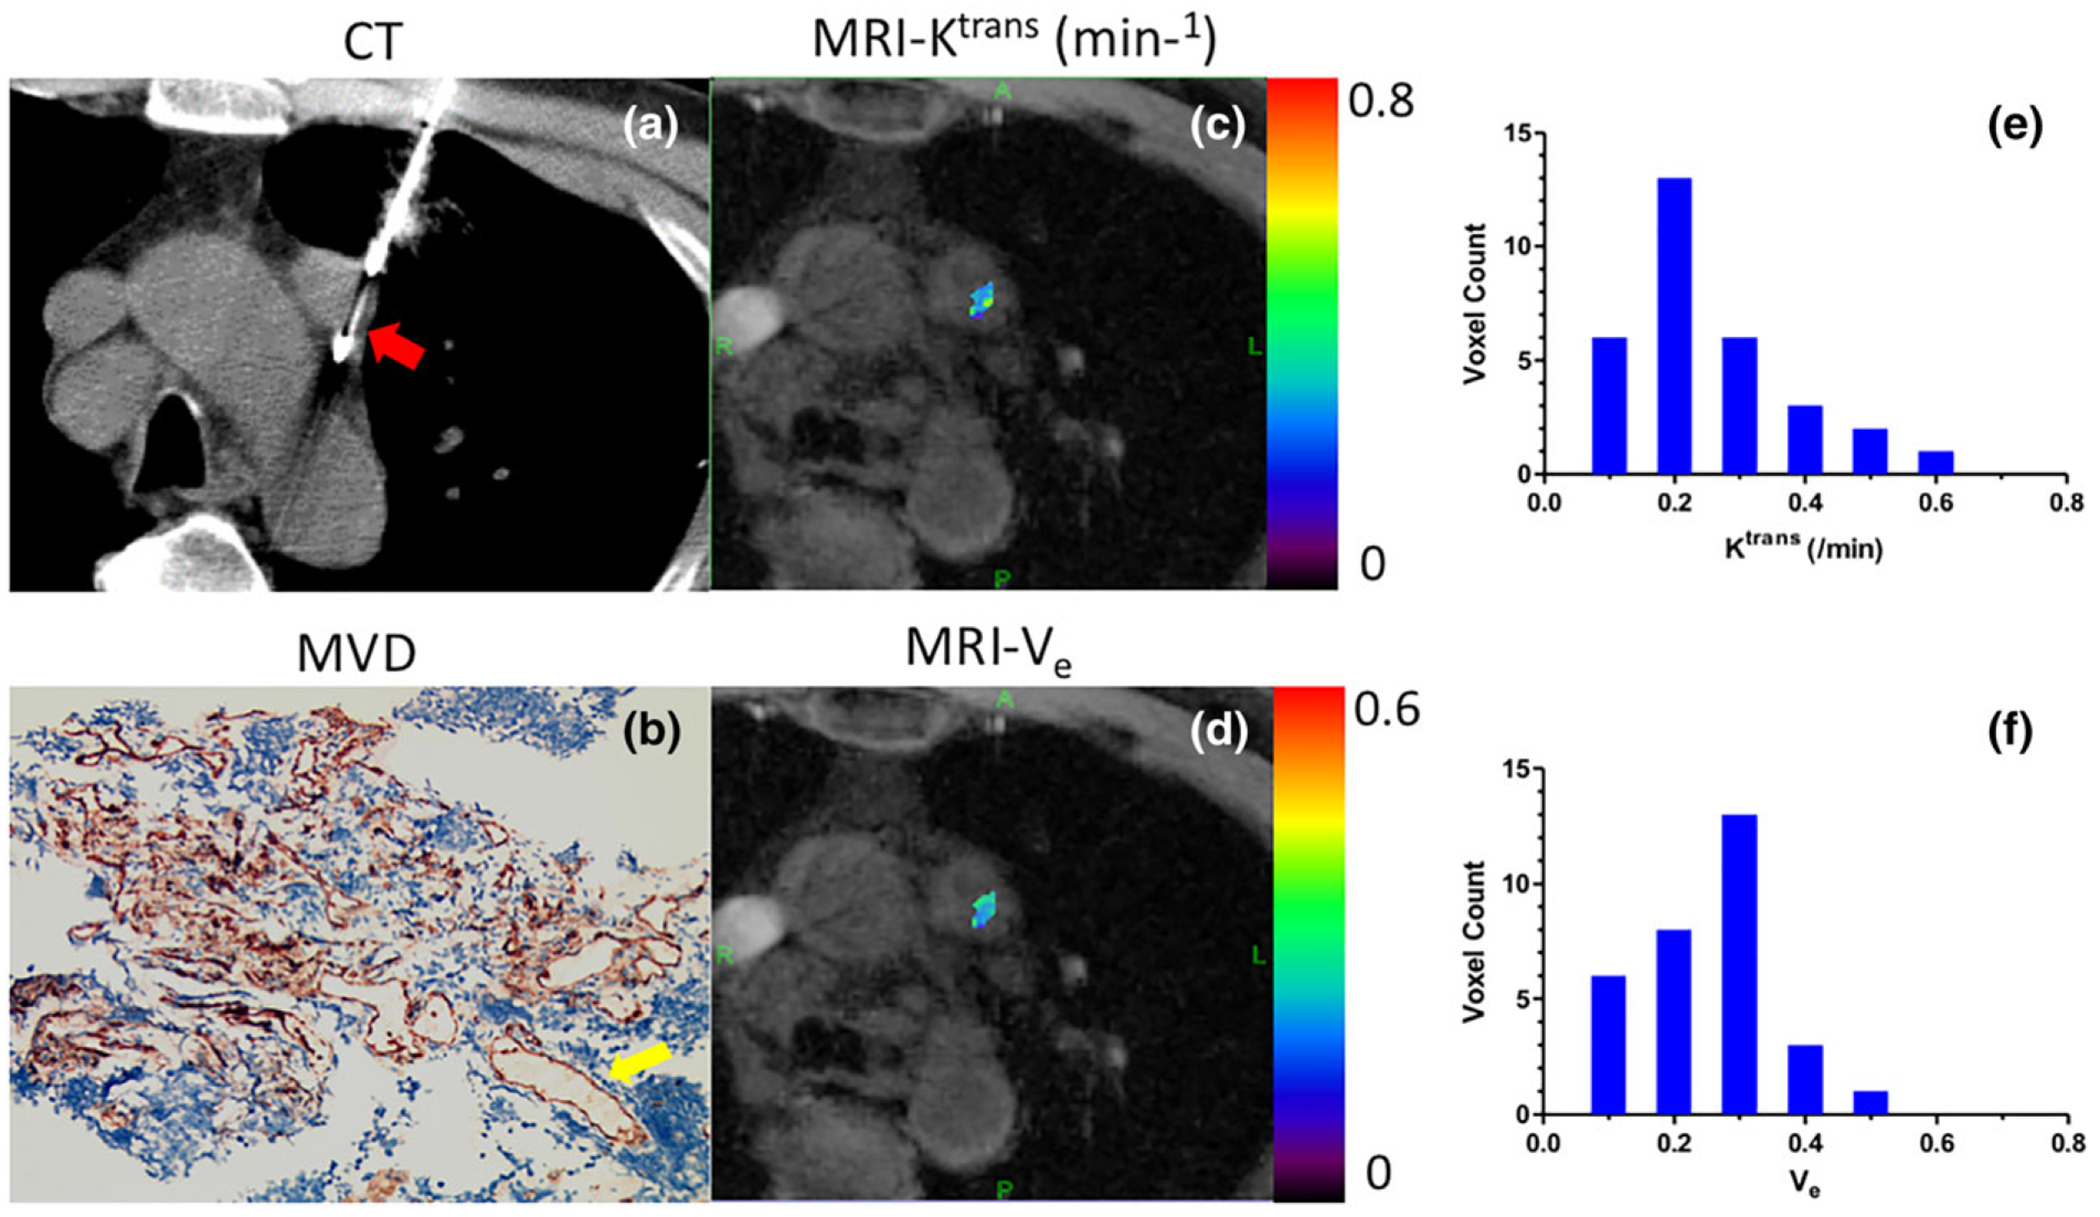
CT-biopsy image (a), MVD image (b), and regional K^trans^ and V_e_ maps superimposed on a corresponding GRASP image (c,d) from a patient with small-cell carcinoma. Corresponding histograms of the K^trans^ and V_e_ maps (e,f) show the variation of the K^trans^ and V_e_ values from pixel to pixel. The yellow arrow indicates a microvessel and the red arrow indicates the needle in CT biopsy.
